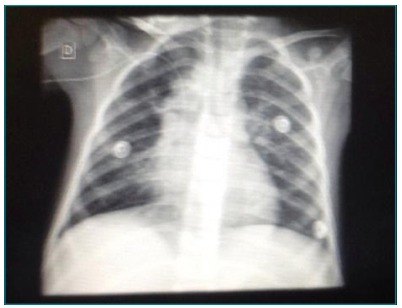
Control X-ray two days after discharge from the Pediatric Intensive
